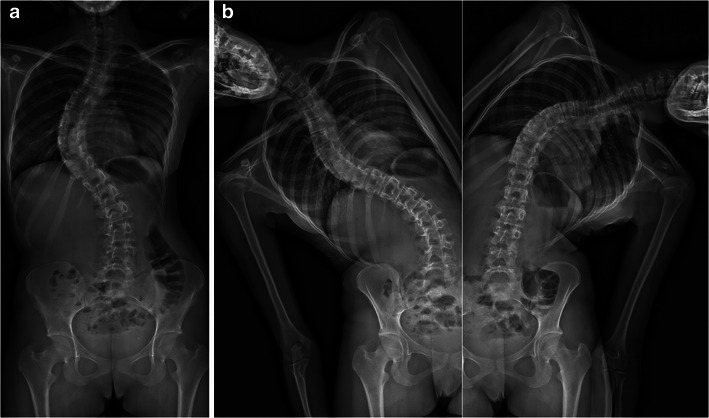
Structural versus non-structural spinal curves in a 15-year-old girl. The AP radiograph reveals right thoracic and left lumbar curvatures (a). On bending radiographs (b), the right thoracic curve (structural curve) is non-correctable, whereas the left lumbar curve (non-structural curve) is correctable with bending to the left. [Total DAP = 864 mGy·cm^2^]
